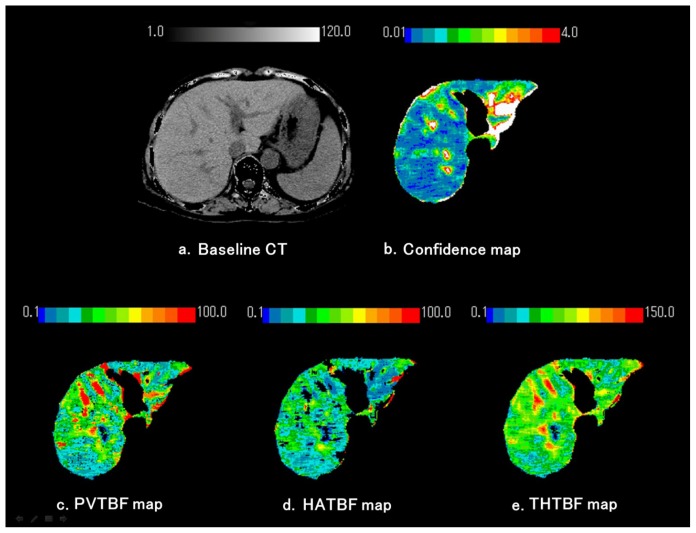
Measurement of hepatic tissue blood flows (TBFs) and confidence values obtained using xenon computed tomography (Xe-CT). Maps were created for portal venous TBF (PVTBF; c), hepatic arterial TBF (HATBF; d), the Xe solubility coefficient, and confidence values for each pixel in the liver, on the basis of changes over time in the Xe-CT numbers in hepatic tissue and spleen. (a) Baseline CT; (b) Confidence map. The original blood flow maps were modified by automatically excluding any pixels with confidence values exceeding the threshold in the confidence map. The white areas on the confidence map indicate regions of low reliability and were automatically excluded. Confidence values indicate the difference between theoretical and actual changes over time on Xe-CT; (c) Portal tissue blood flow (PVTBF) map; (d) Hepatic arterial tissue blood flow (HATBF) map; (e) Total hepatic tissue blood flow (THTBF) map.
